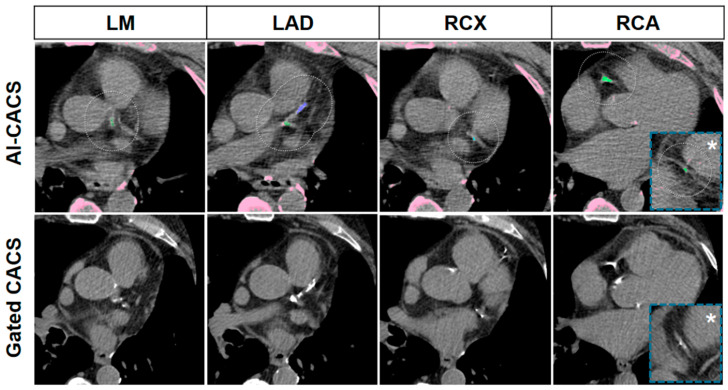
Representative CT images of a 69-year-old man with a body mass index of 26.3 kg/m^2^ with severe coronary artery calcifications. Images from ungated CT from a PET/CT performed for restaging of a rectal adenocarcinoma are presented in the upper row, and dedicated gated CAC CT from myocardial perfusion single photon emission computed tomography performed 99 days later are presented in the lower row. Coronary calcifications in the left main (LM), left anterior descending (LAD), ramus circumflexus (RCX), and right coronary artery (RCA) including the distal segment (asterisk), were correctly marked by the AI-CACS tool resulting in a score of 865. The score from the dedicated CAC scan was 942.
